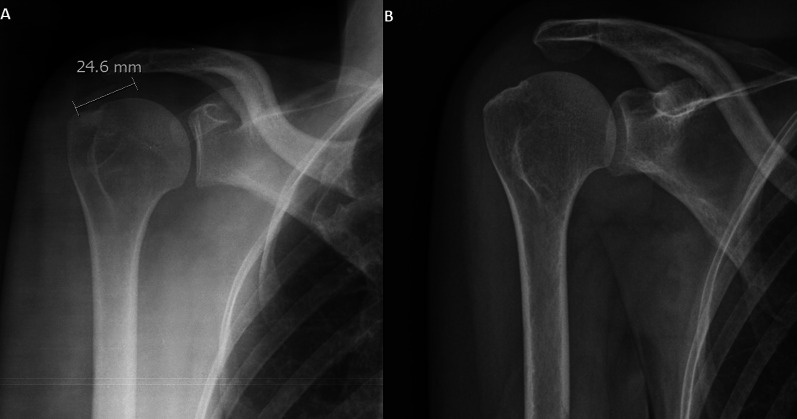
Anteroposterior shoulder x-ray performed at baseline (A) and after 10 years (B) shows complete resorption of the calcification
